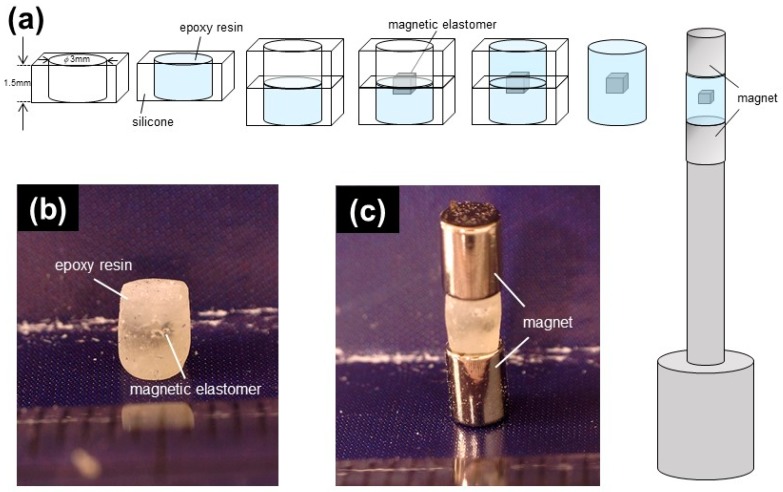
(a) Schemes of sample preparation for CT observations; a piece of magnetic elastomer was placed in epoxy resin. Photos of samples at (b) 0 mT and (c) 270 mT; sample was sandwiched by two permanent magnets with a magnetic field of 490 mT.
